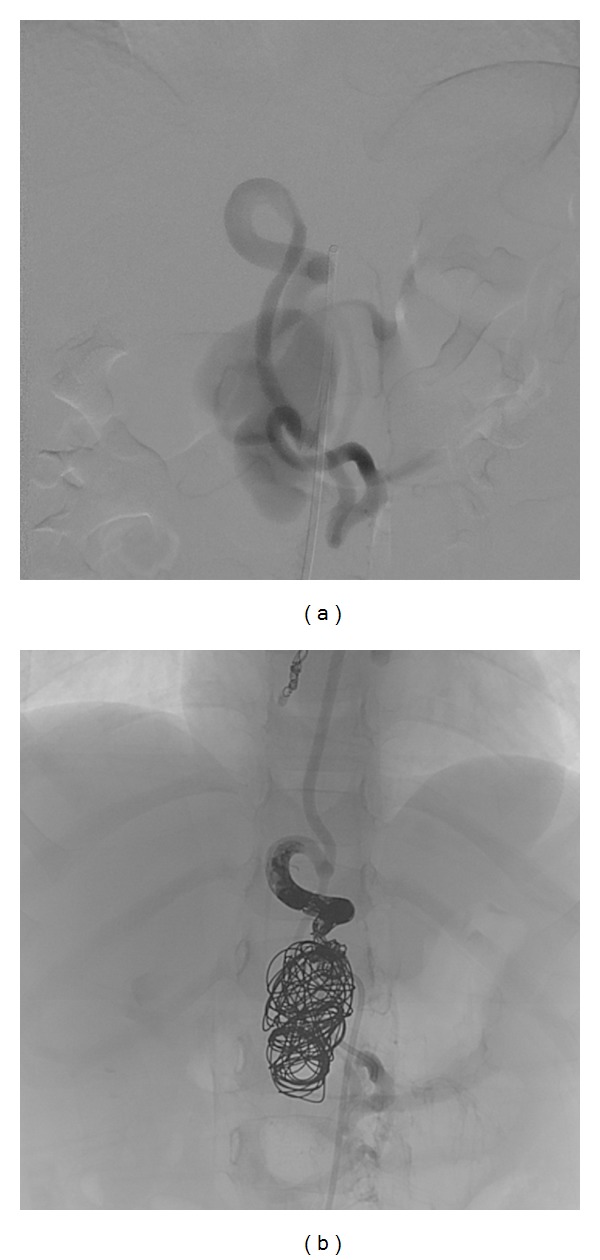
Angiographic imaging: (a) spinal angiogram, arterial phase, anteroposterior view, shows the arteriovenous fistula and the giant aneurysm at the level of T12/L1; (b) X-ray, AP view, same level, shows the coils and Onyx cast after endovascular occlusion.
